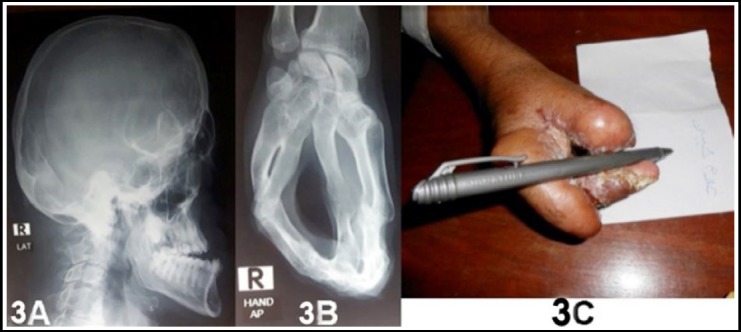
3A: X-Ray Skull. 3B: X-Ray of the hand. 3C: Right hand after the operation.
